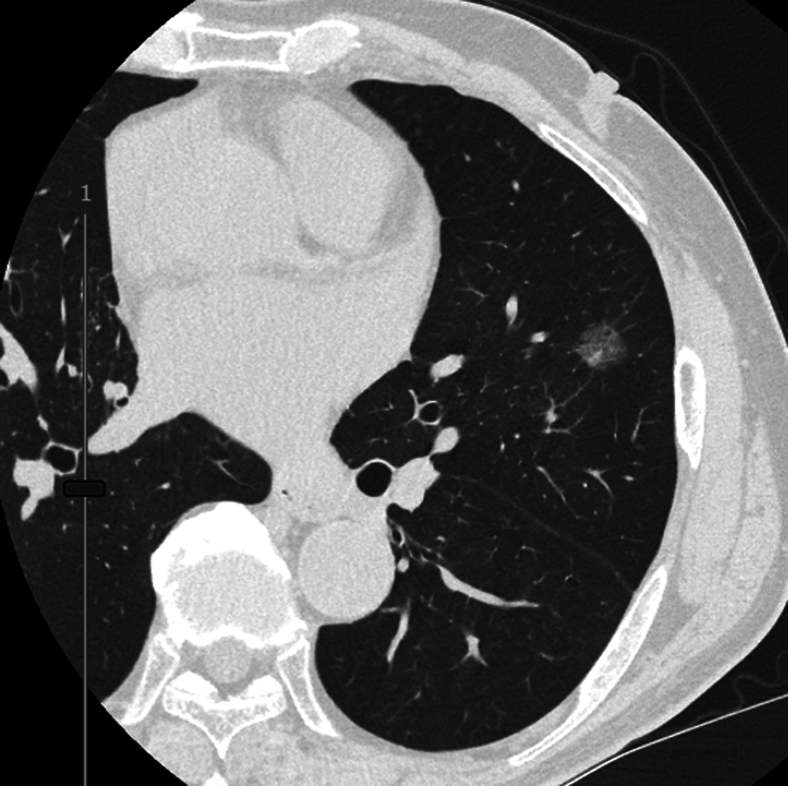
A computed tomography showing the target lung cancer in the left lingula.
